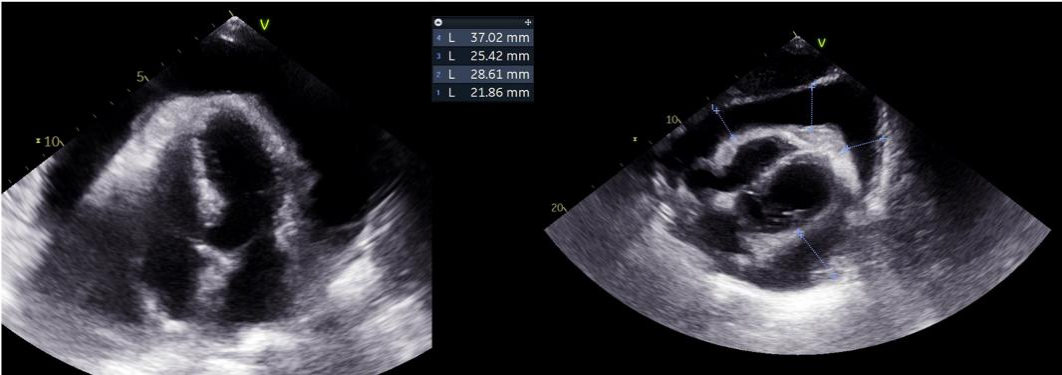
Pericardial effusion (transthoracic echocardiogram).
